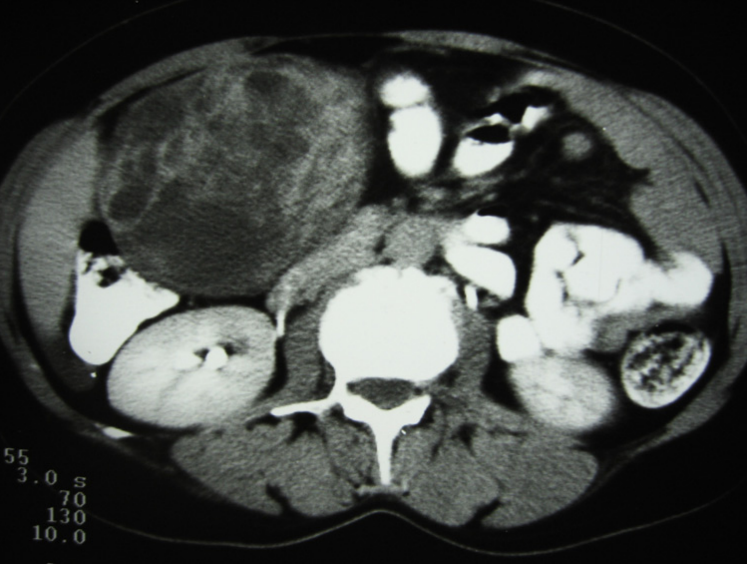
Contrast-enhanced abdominal CT scan, with oral and i.v. contrast, shows a large (15 × 15 cm), well-demarcated, solid and cystic, heterogeneously enhanced mass located between the right lobe of the liver and the right kidney.
